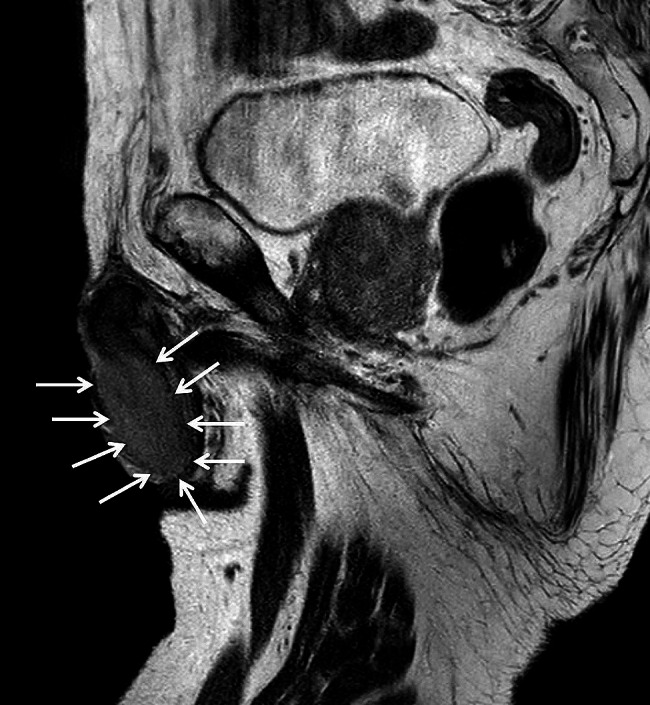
Magnetic resonance imaging of the glans penile tumor. A T2-weighted image revealed a large soft tissue mass in the glans penis and corpus cavernosum (white arrows)
